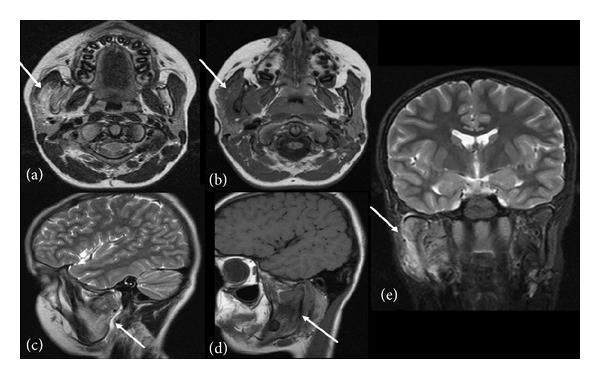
(a) T2-weighted axial 3 mm spin-echo sequences. (b) T1-weighted axial 3 mm spin-echo sequences. (c) T2-weighted sagittal 4 mm turbo spin-echo sequences. (d) T1-weighted sagittal 3 mm turbo spin-echo sequences. (e) T2-weighted coronal 4 mm turbo inversion recovery magnitude sequences. (a)–(e) MRI sequences reveal enlarged mandibular bone structures with a loss of regular bony cortex and a soft tissue mass infiltrating the temporal and masseter muscles.
